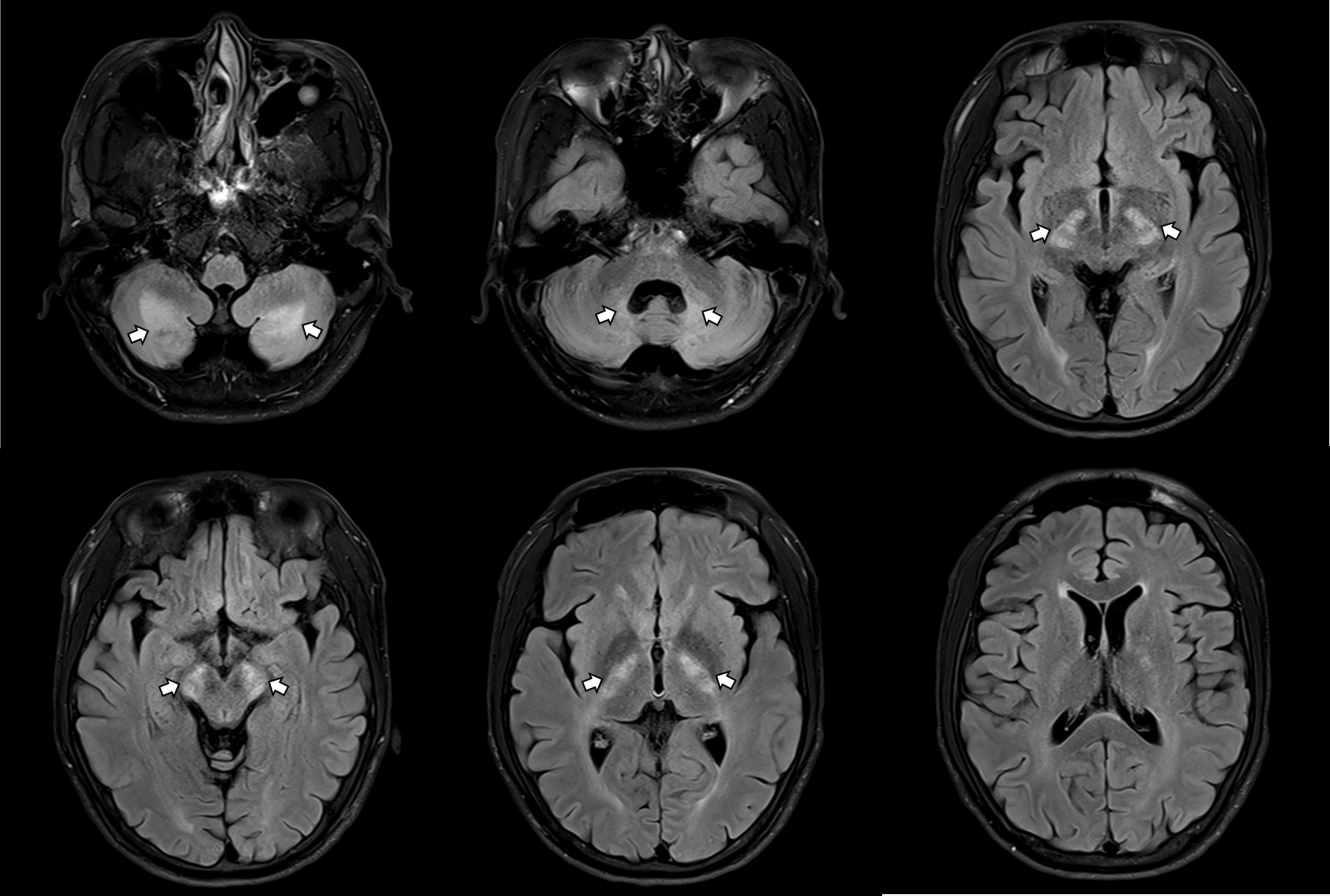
Brain MRI revealing symmetrical abnormalities in multiple brain regions.
